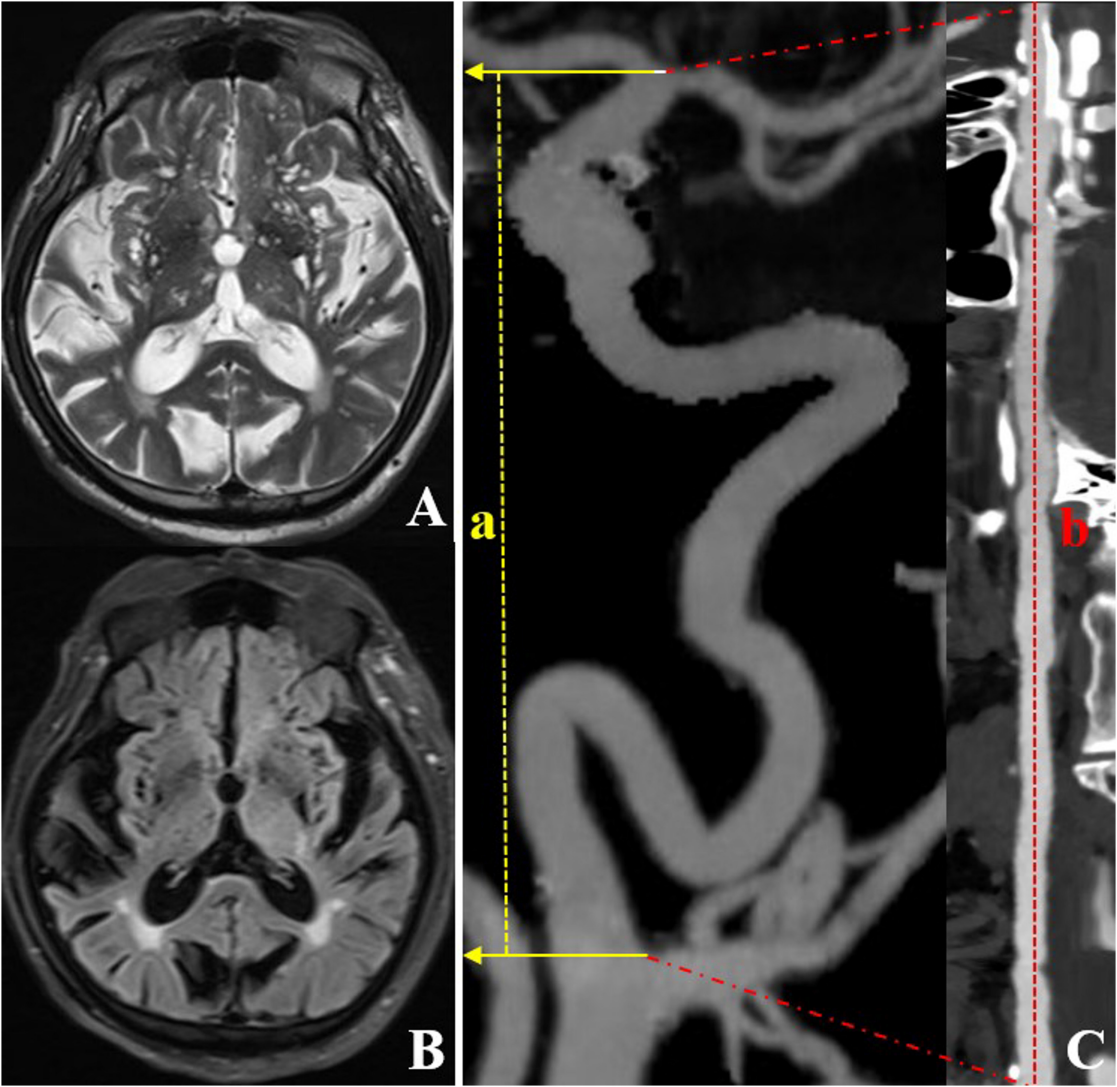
CTA and MRI images of an individual (a 72-year-old woman) with left ICA TI equal to 2.64. T2WI (A) and FLAIR (B) showed a grade 4 EPVS in the basal ganglia region. CTA with MIP (C) shows severe tortuosity of the left ICA. The dotted line a represents the straight length of the ICA, and the dotted line b represents the centerline vessel length of ICA reconstructed by using curved planar reformat. CTA, computed tomography angiography; EPVS, enlarged perivascular spaces; ICA, internal carotid artery; MIP, maximum intensity projection; MRI, magnetic resonance imaging; TI, tortuosity index.
